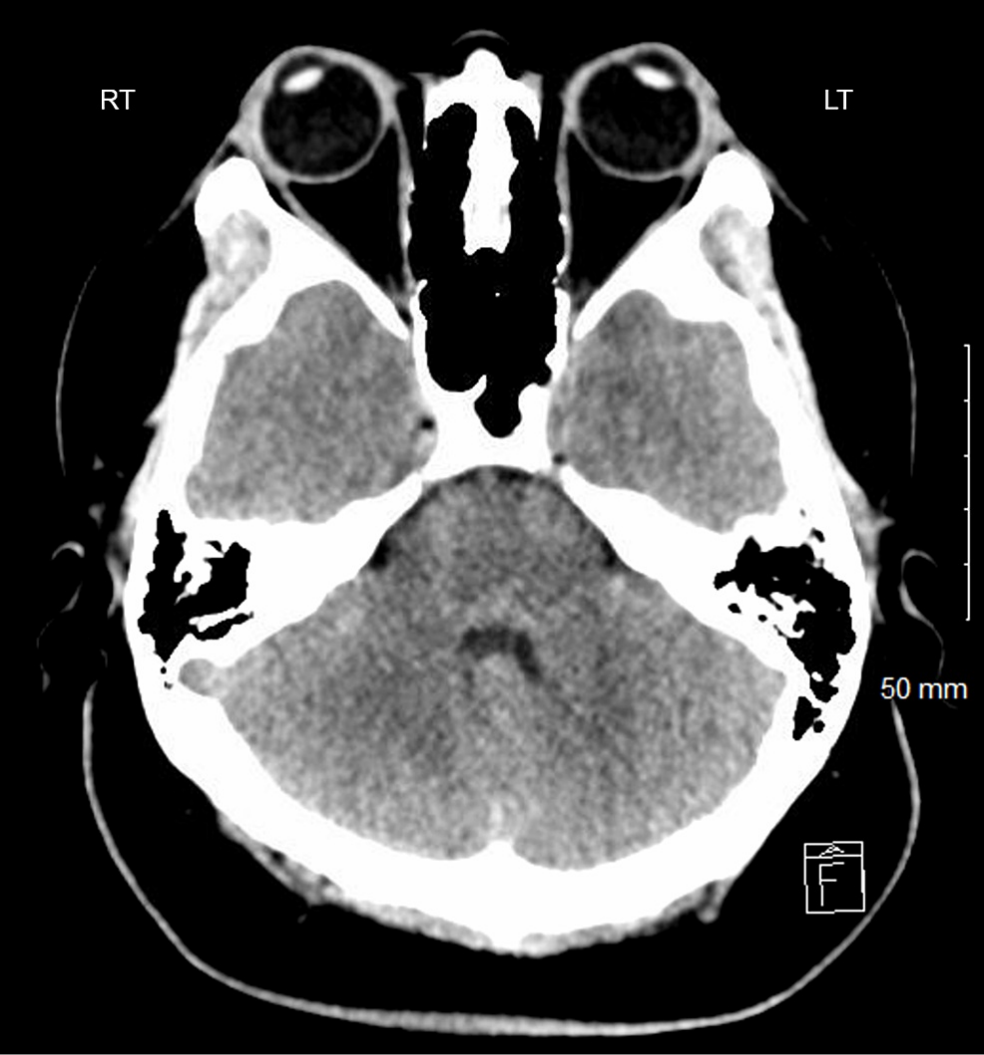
Normal axial CT scan at the level of the fourth ventricle
